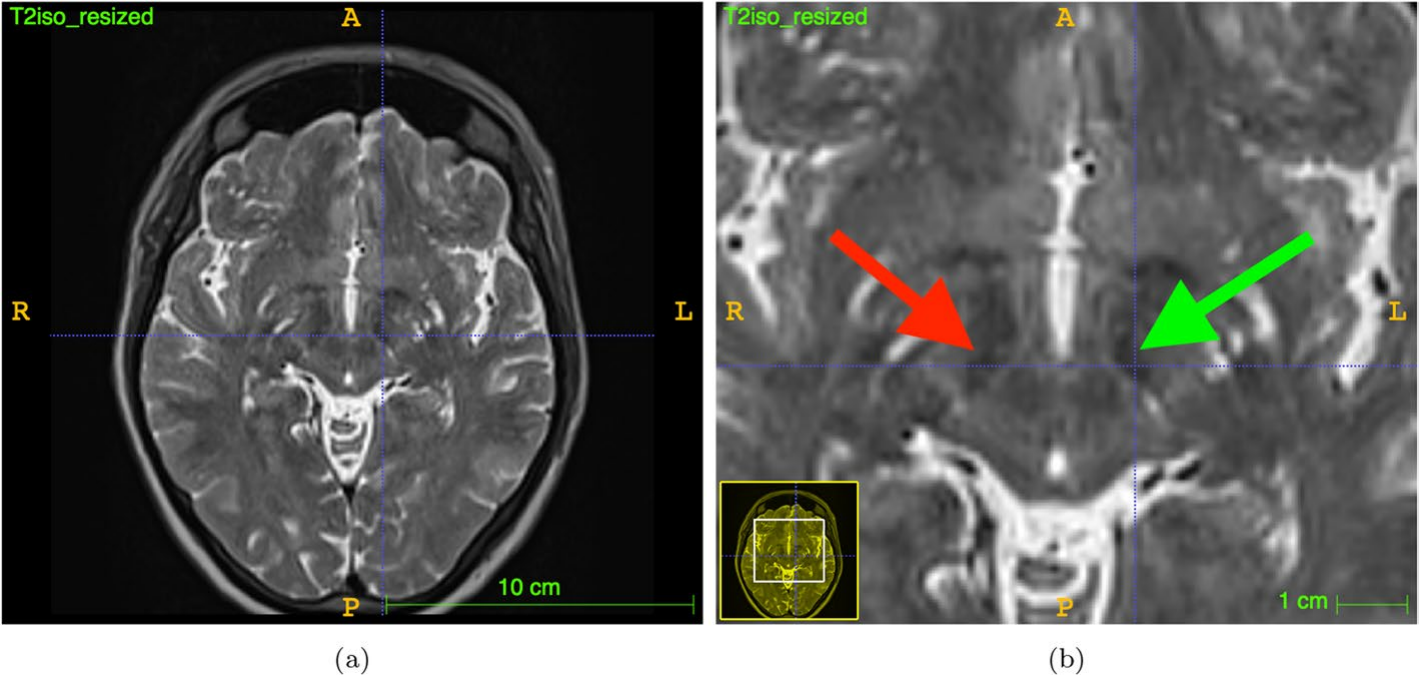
a Preoperative T2-weighted MRI, and b characteristic hypointensities depicting right and left STN on preoperative T2-weighted MRI (right: red arrow, left: green arrow)
